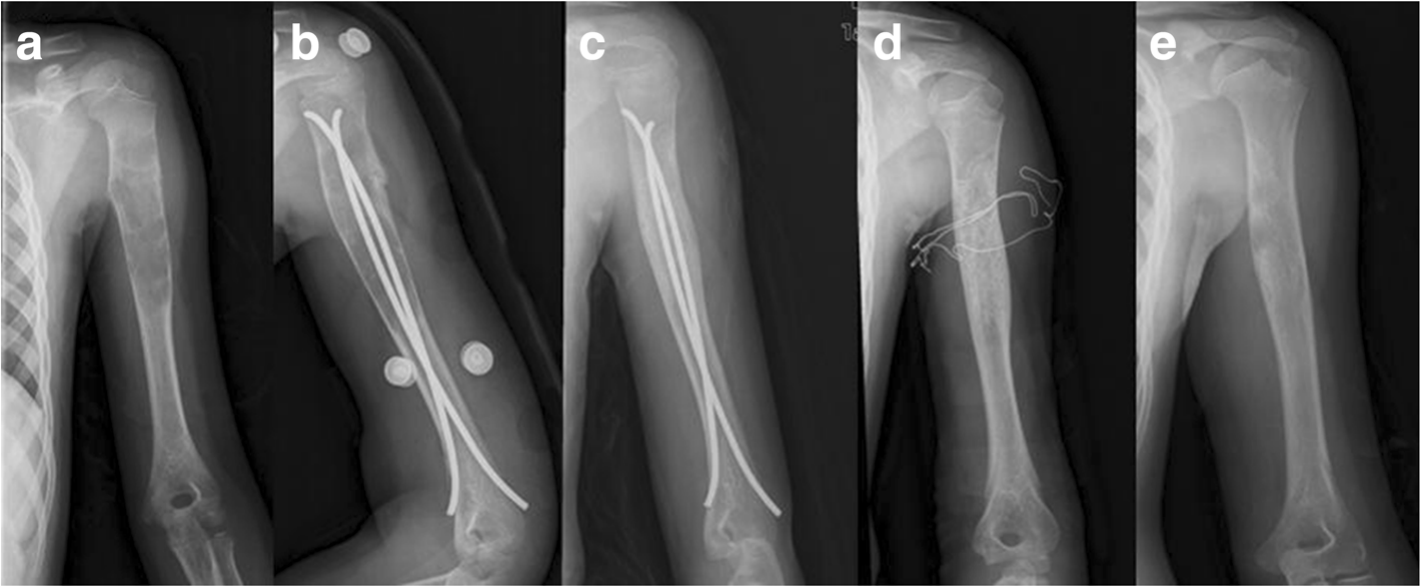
Radiographs of an 8-year-old girl who presented with pathological fracture of the right humerus. a Anteroposterior radiograph at presentation. A bone cyst with pathological fracture is located in the proximal metaphysis and diaphysis of the humerus. b Fixation with flexible intramedullary nails was performed. c Three months after surgery the lesion is significantly smaller and shows sings of healing. d At 28 months the bone cyst has resolved, and the intramedullary nails are removed. e At 61-month follow-up the bone cyst is completely healed without refracture or deviation of the axis
